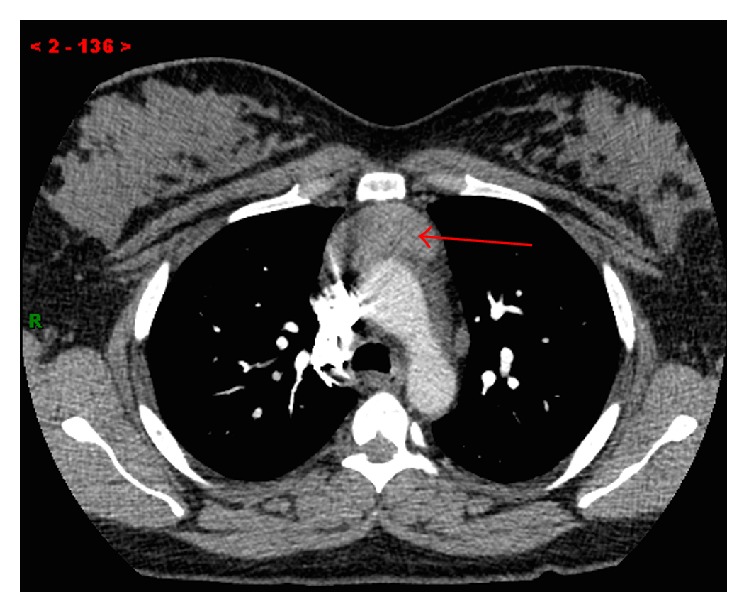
Axial CTA through the level of the aortic arch shows a homogenous anterior mediastinal mass (red arrow).
